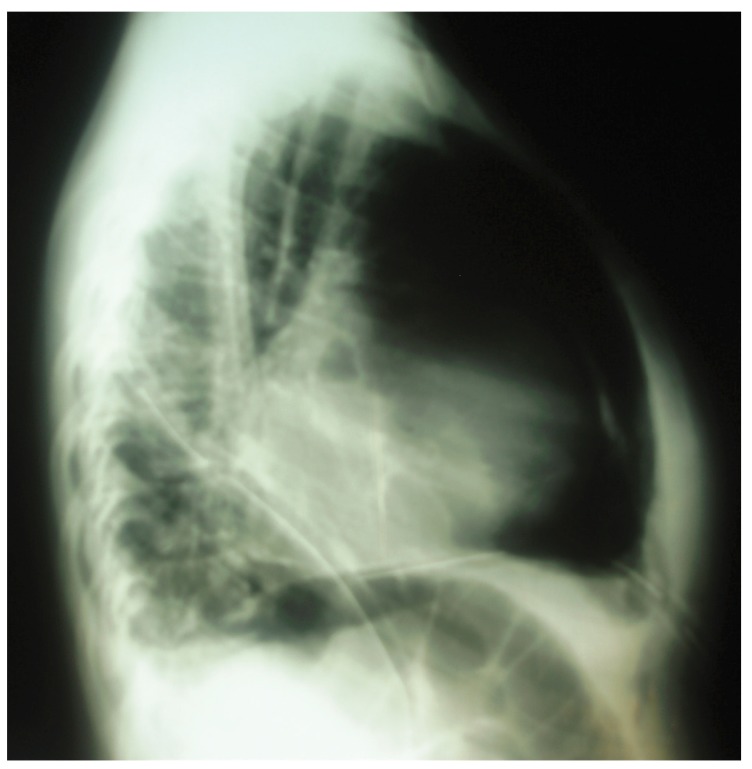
Radiograph showing evidence of pericardial calcification.
